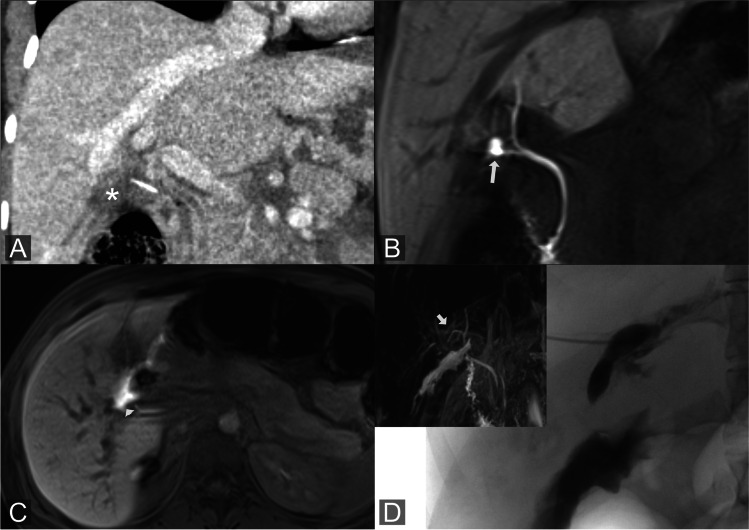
Central bile leakage: CT of a 42-year-old patient with a small subhepatic postoperative biloma (asterisk) after laparoscopic cholecystectomy (A). Gd-EOB-DTPA-MRCP in coronal plane shows extraluminal Gd-EOB-DTPA at the central right hepatic duct (arrow) with suspected injury (B). Axial plane depicts the extensive Gd-EOB-DTPA extravasation at the liver hilum (arrow head) (C). After unsuccessful ERCP, percutaneous cholangiogram of a right segmental duct confirms the high volume, central leakage of the right main hepatic duct (left corner: T2w-MRCP shows the anatomy of the whole biliary tree; arrow: entry point of the PTC canula). The extrahepatic common hepatic duct could not be cannulated across the large defect, so that no sufficient drainage could be achieved. Ultimately, this patient had to undergo surgical revision with creation of a biliodigestive anastomosis (D)
